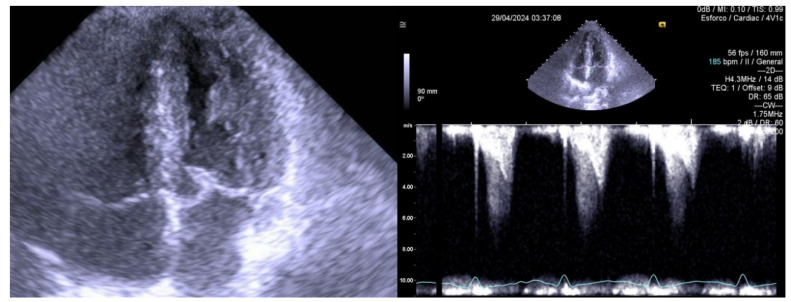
Peak exercise stress echocardiography off beta-blocker, with SAM (systolic anterior movement of the mitral valve (Left)), with intraventricular gradient (right), and with angina pectoris.
